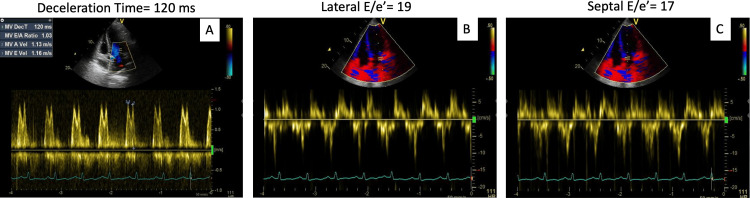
Echocardiogram, apical 4-chamber view with diastolic evaluation Echocardiogram Apical 4 chamber view with pulse wave tissue Doppler imaging showing evidence of elevated left ventricular filling pressures, diagnostic of congestive heart failure. A) Mitral inflow pattern with a deceleration time. B) Lateral E’ velocity C) Septal E’ velocity
